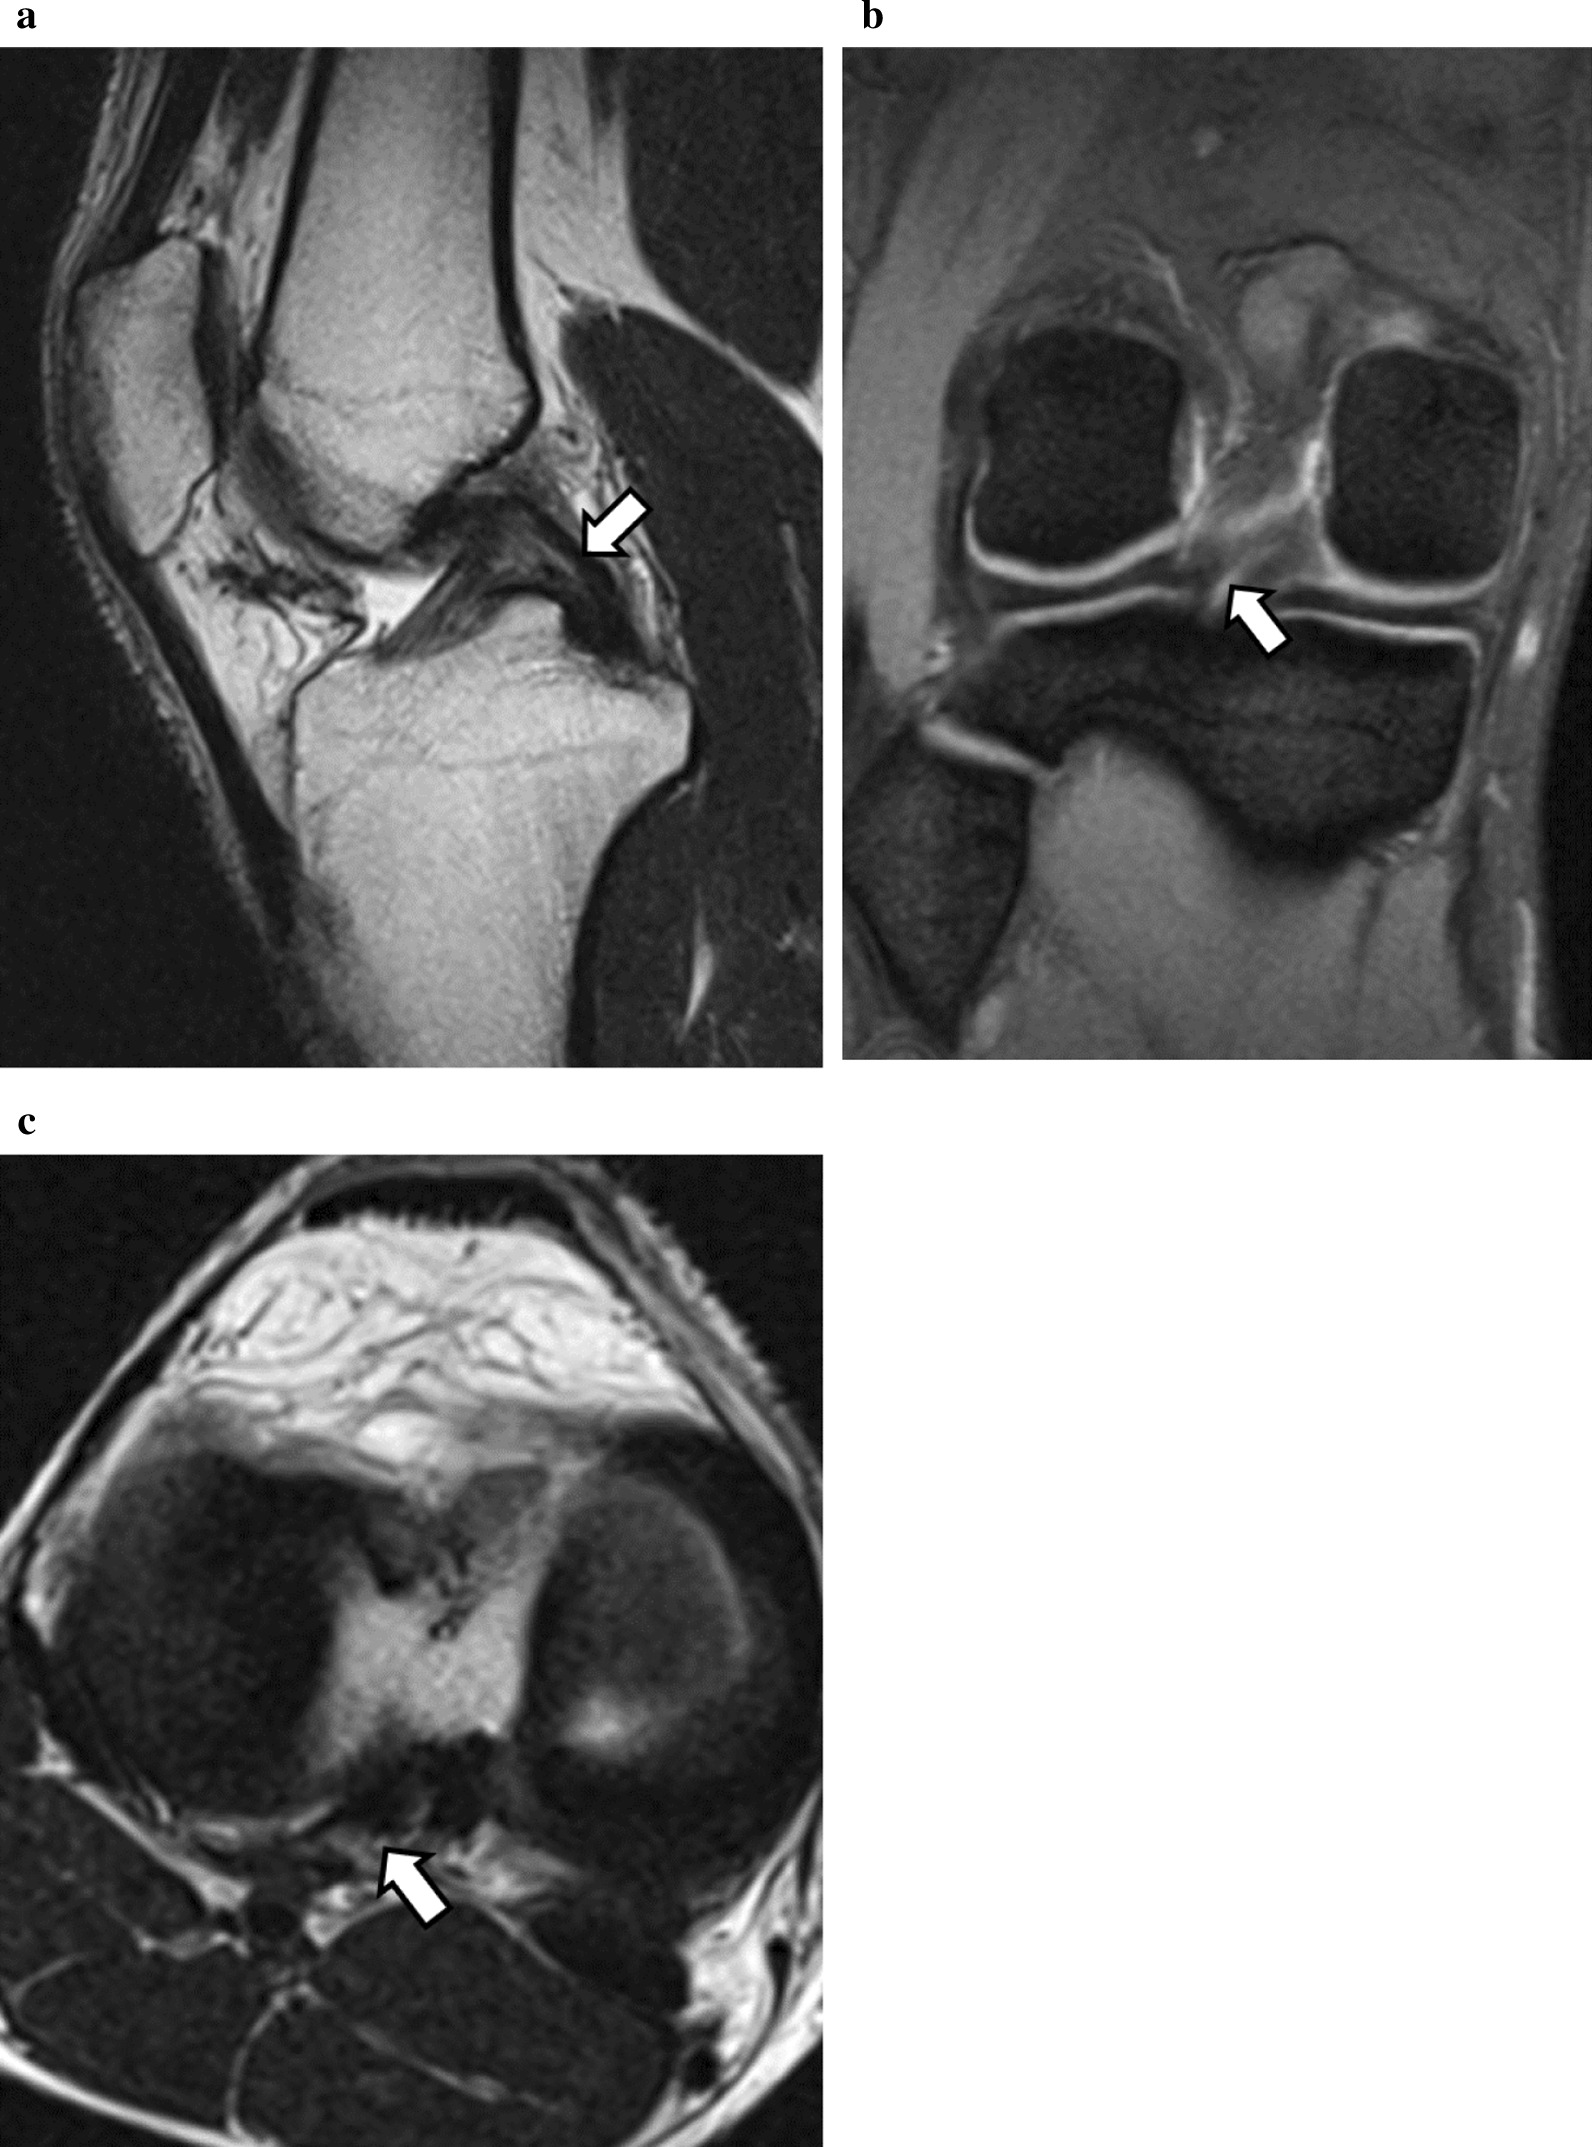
Postoperative MRI at 12 months after surgery. At 12 months postoperative sagittal (a), coronal (b), and axial (c) T2-weighted MRIs showed no evidence of recurrence and indicated that the remaining PCL was thicker than before the surgery (white arrow)
